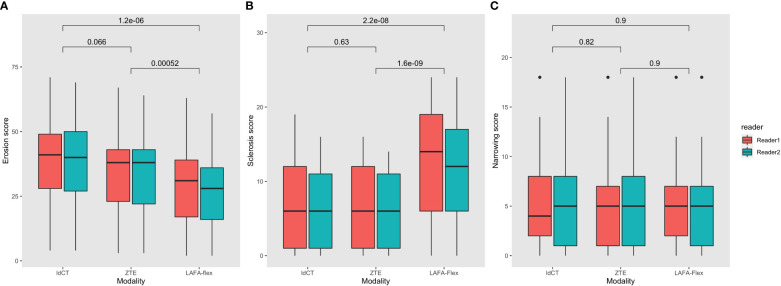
Comparison of sum scores of structural lesions of the sacroiliac joint with ldCT, ZTE and LAVA-Flex. (A) Erosions; (B) Sclerosis; (C) Joint space changes. ldCT, low-dose CT; ZTE, Zero echo time; LAVA-Flex, Liver Acquisition with Volume Acceleration-flexible.
